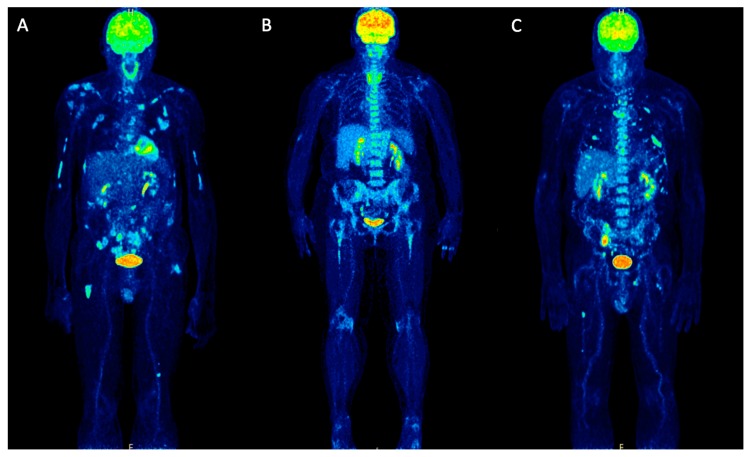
Maximum intensity projection (MIP) PET/CT images of newly diagnosed MM patients before treatment, representing examples of different pathologic patterns of ^18^F-FDG uptake. (A) demonstrates a patient with multiple focal lesions in the skeleton. (B) depicts a patient with intense diffuse tracer uptake in the bone marrow of the axial skeleton and the proximal humeri and femora without clearly delineated focal lesions. (C) shows a patient with a mixed pattern of ^18^F-FDG uptake with intense, diffuse uptake in the axial skeleton and multiple, focal bone marrow lesions.
